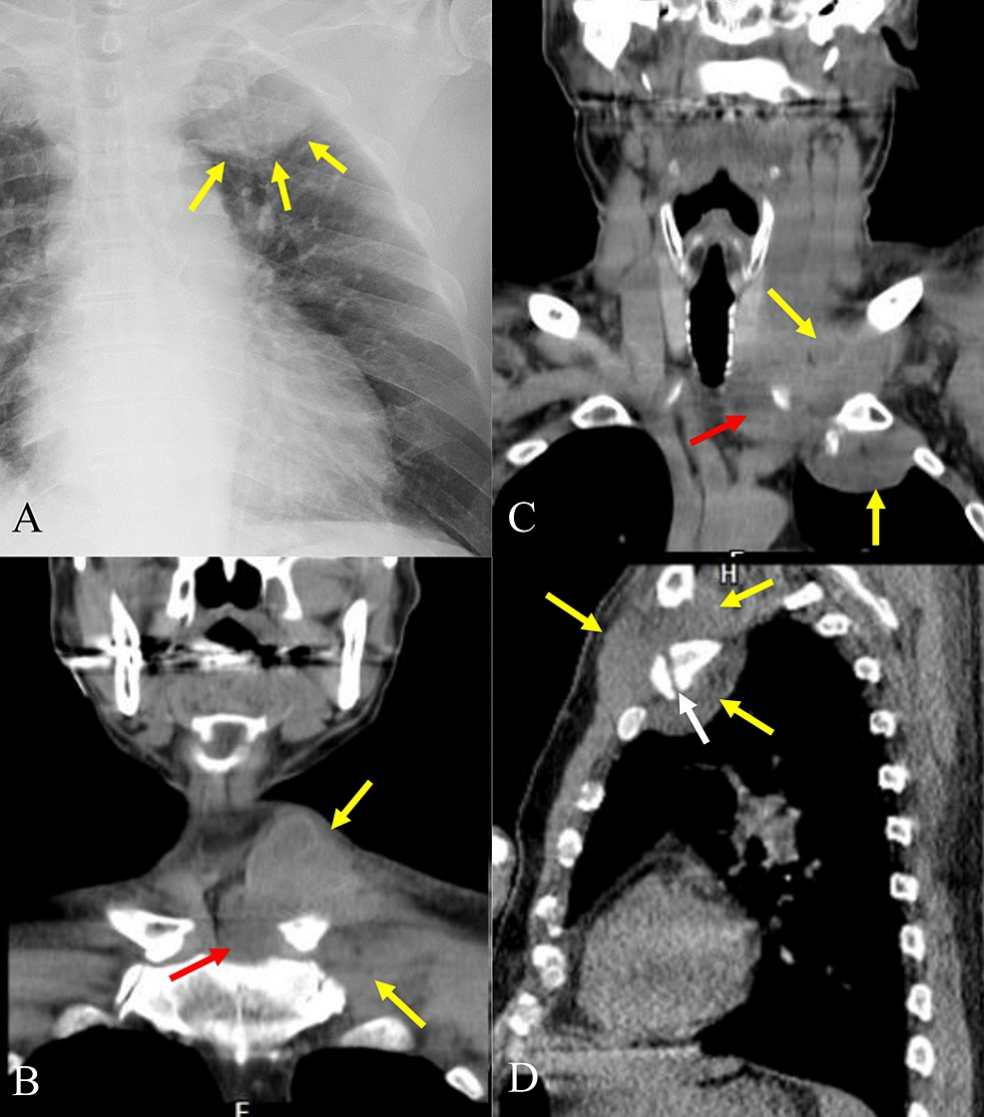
Initial chest roentgenogram and computed tomography. A: Initial chest roentgenogram showing homogeneous density in the left chest apex (yellow arrows). B-D: Computed tomography showing a periarticular abscess surrounding the left sternoclavicular joint (yellow arrows). There is abnormal fluid density along the medial aspect of the clavicular head (red arrows), but bone erosion and cortical irregularity were not noted. Widening of the costochondral junction of the 1st rib was demonstrated (white arrow).
